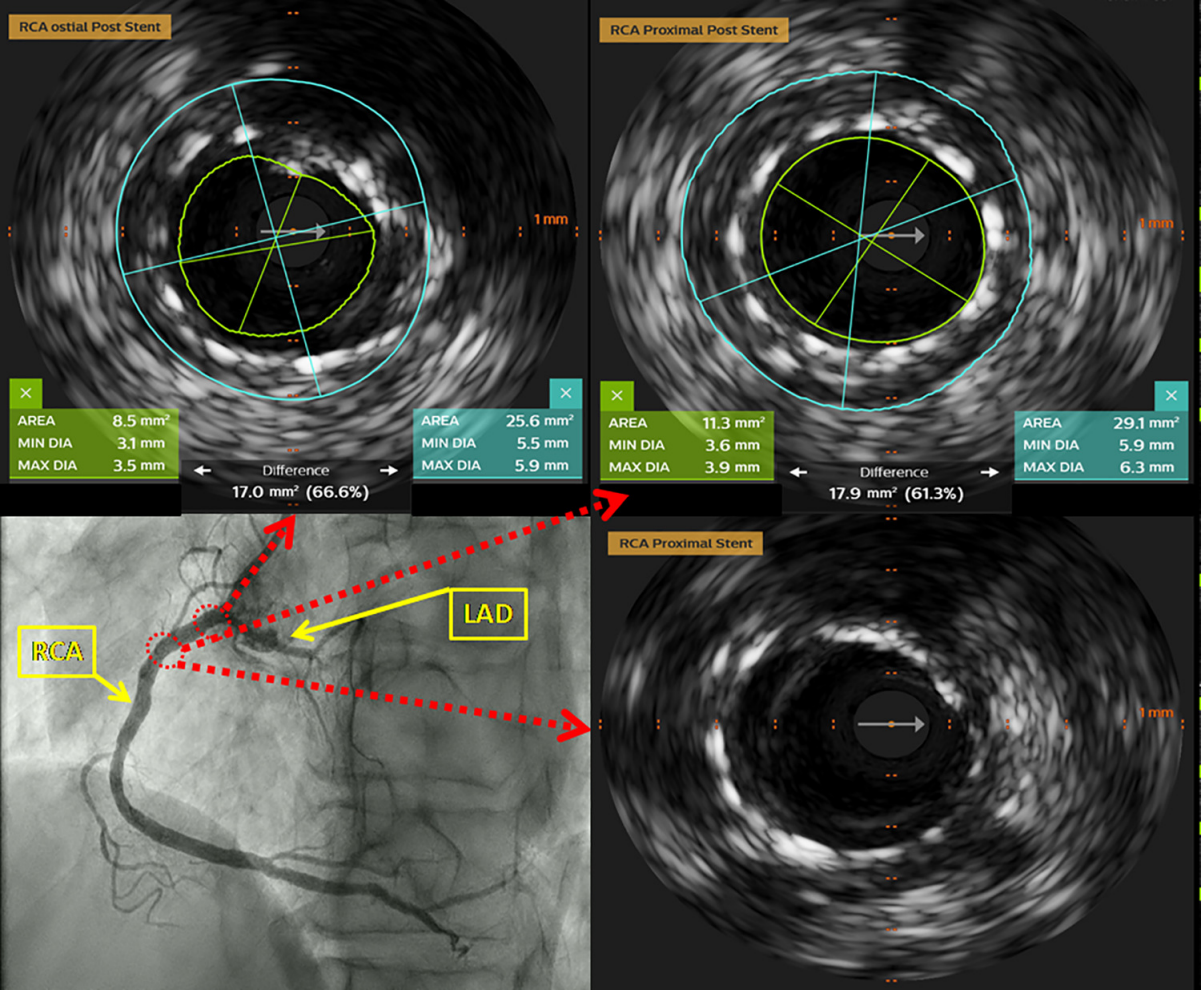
Intravascular ultrasound (IVUS) of the RCA after PCI. The optimal stent apposition without signs of dissection on proximal and distal stent edge was observed.
